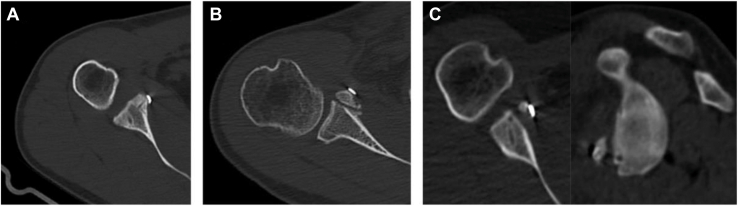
Post-operative CT scan. (A) Complete healing. (B) Partial healing. (C) No healing. *CT*, computed tomography.
